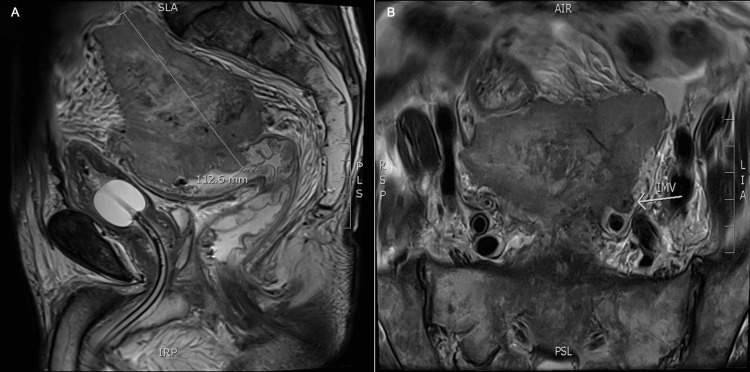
MRI demonstrating a 112-mm rectosigmoid tumor with direct invasion of the presacral fascia above the anterior peritoneal reflection, direct invasion of IMV, and a 5-mm left obturator lymph node (A = sagittal view, B = axial view). MRI, magnetic resonance imaging; IMV, inferior mesenteric vein
